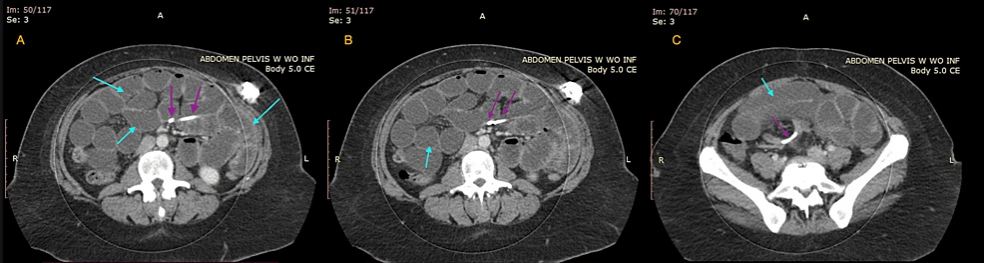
Axial non-contrast abdominal CT demonstrating dilated small bowel loops Non-contrast CT of the abdomen in the axial plane (A-C) demonstrating multiple, dilated small bowel loops (blue arrows), along with the path taken by the LAGB connecting tube to intertwine with mesenteric circulation (purple arrows) LAGB: laparoscopic adjustable gastric banding
